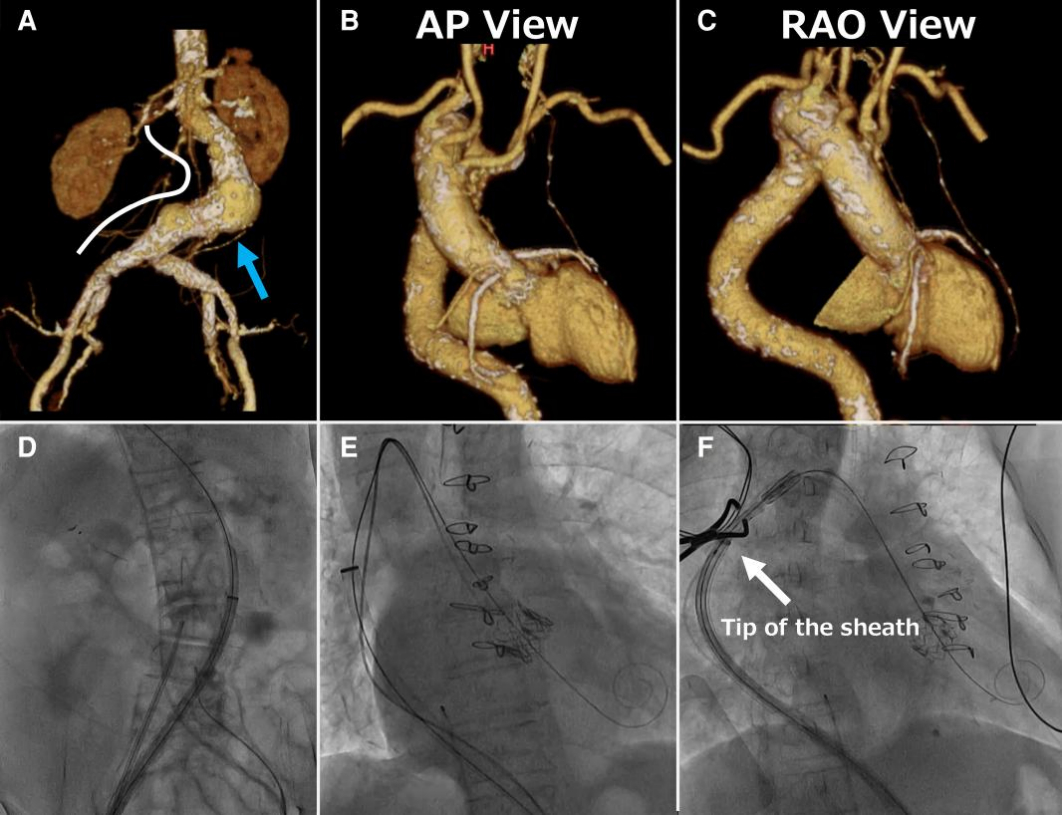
A and D: (*A*) CT volume rendering showing an abdominal aortic aneurysm (arrow) with severe tortuosity and (*D*) the corresponding fluoroscopic view. B and E: (*B*) Anteroposterior view of CT volume rendering and (*E*) the corresponding fluoroscopic view. C and F: (*D*) Right anterior oblique view of the CT volume rendering and (*F*) corresponding fluoroscopic view. CT, Computed tomography.
